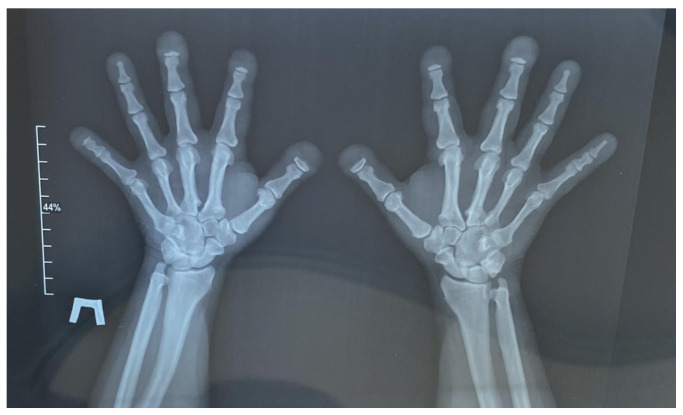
Radiograph of the hands in two projections. Acro-osteolysis with a predominant lesion of the distal phalanges of the 1st, 2nd, 3rd, and 5th digits on the right and the 1st, 2nd, and 3rd digits on the left by the type of nail tubercle resorption is noted. The small tubular bones of the hands are shortened. The interphalangeal and metacarpophalangeal joints are not constricted, and the articular surfaces have clear, even contours. Elongation of the ulnar styloid processes is noted. Para-articular tissues are not altered. There is no evidence of bone destruction.
